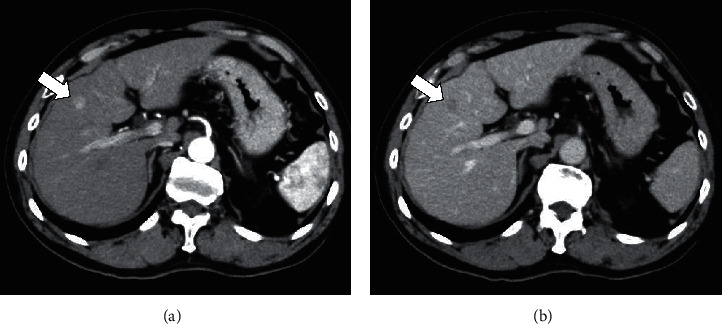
Contrast-enhanced CT showing the tumor (a) markedly enhanced in the arterial phase (b) followed by a relatively quick wash-out during the equilibrium phase (arrow).
